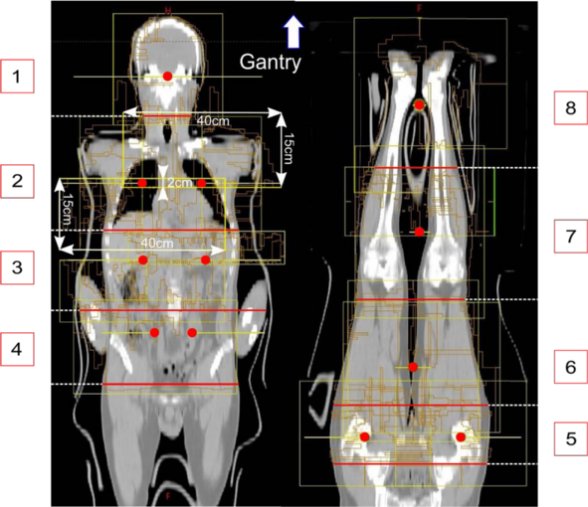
Field (*yellow rectangulars*) and iso-centre (*red dots*) arrangement: for the treatment planning the CT image set is divided into 8 segments (Seg1–8, indicated by the *red lines* and the numbers), with one iso-centre in segments 1, 6, 7 and 8, and with two coplanar iso-centres in segments 2 to 5. In segment 2, the field dimensions for 90° collimator rotation are indicated
